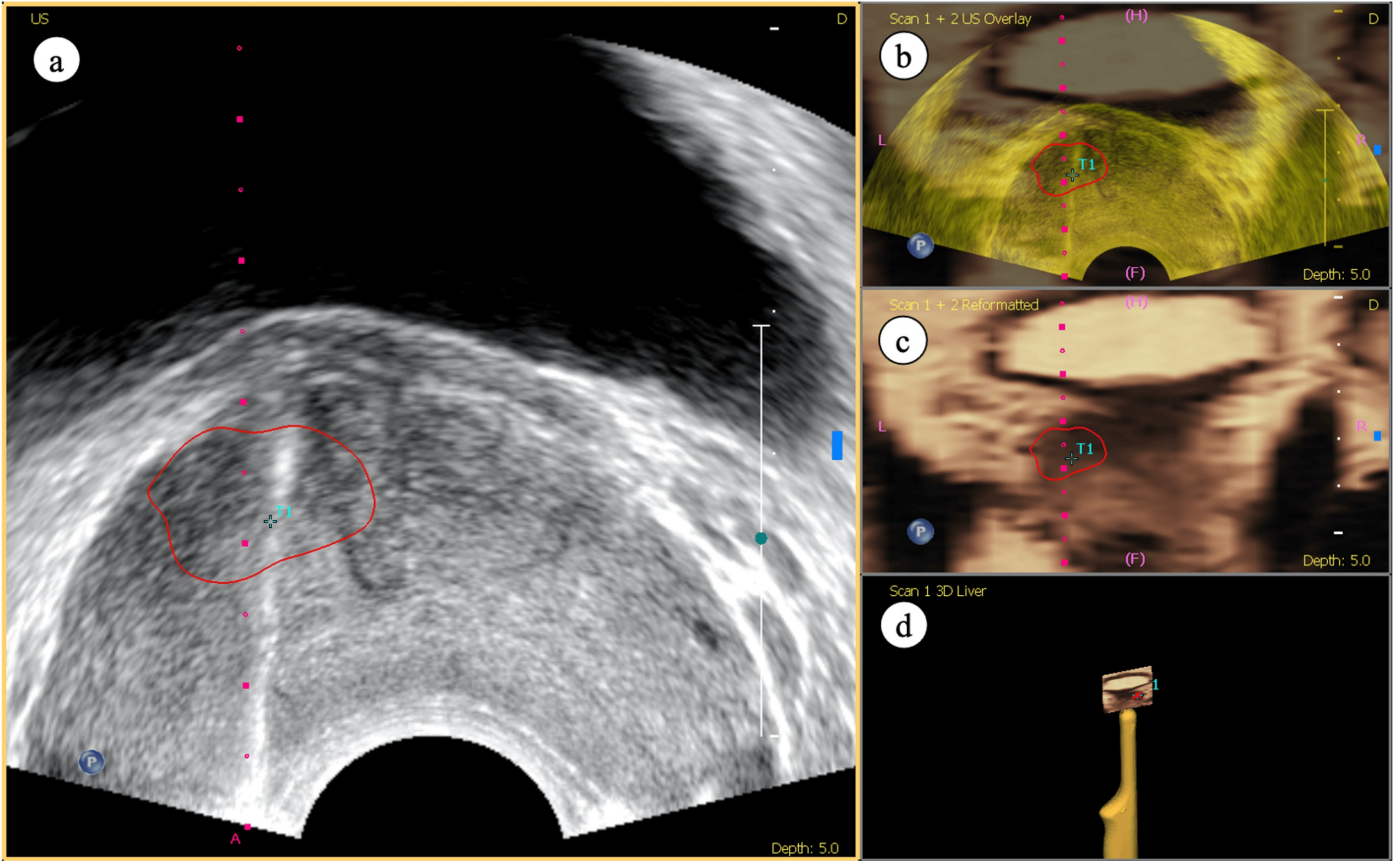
Biopsy of targeted prostate lesion under MRI/TRUS fusion guidance Biopsy needle is seen within the targeted lesion indicated by the red marking, as shown in (a) TRUS image, (b) blend overlay of both ultrasound and MR images, and (c) MR image; (d) reconstructed three dimensional image according to real-time probe orientation. Abbreviations: MRI=magnetic resonance imaging; TRUS=transrectal ultrasound
